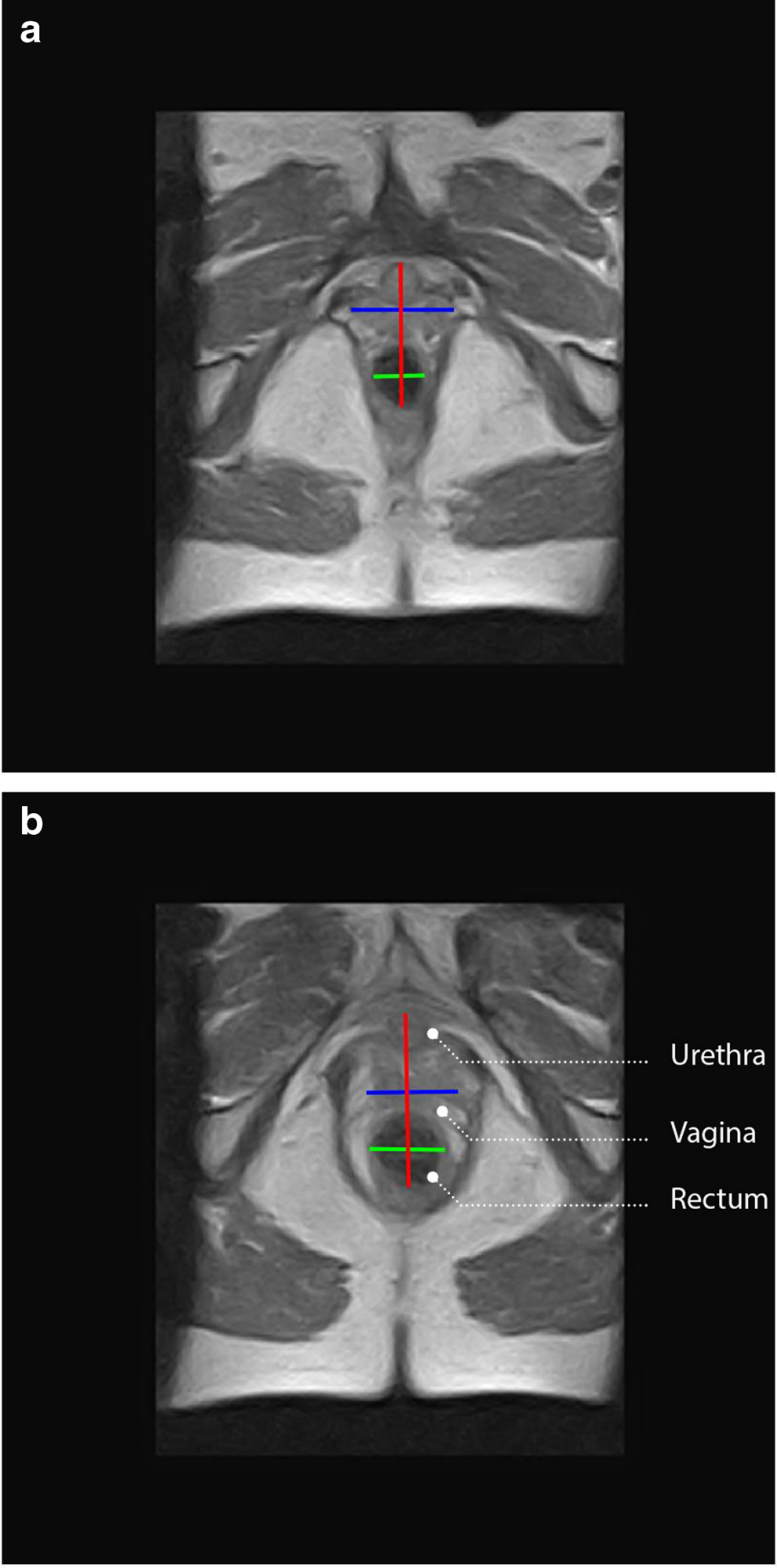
transverse MRI scan of a patient in a the supine–rest and b the upright–rest position. The *blue and green lines* represent the transverse diameter of the genital hiatus. The *red line* represents the anterior–posterior cross-section
